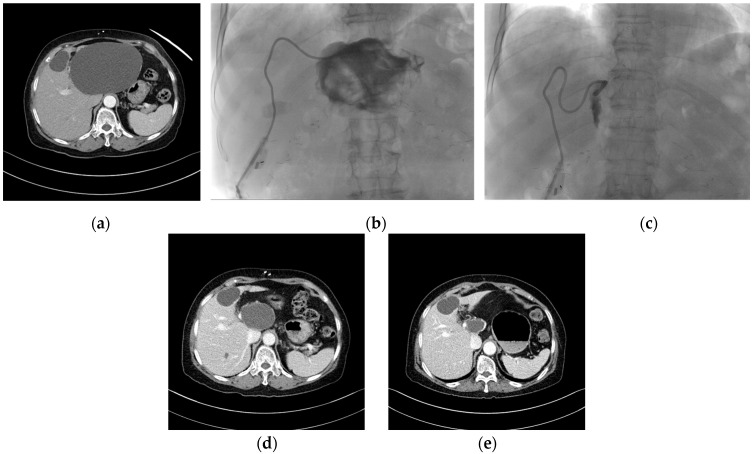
Case of incomplete alcohol sclerotherapy and natural course after treatment. (a) An enhanced CT scan performed on a 77-year-old woman presenting with early satiety revealed a large cyst measuring 14 × 10 × 13 cm maximum orthogonal cyst diameter in the left liver. The patient had a history of alcohol sensitivity. (b) After natural drainage of 1000 mL over the course of one day, tubography was performed. The cystic cavity was found to be collapsed, and contrast infusion and regurgitation proceeded smoothly. The planned injection of 100 mL of 99% alcohol was then initiated. However, at the time of injection of 40 mL, severe symptoms of alcohol intoxication manifested, which led to an immediate discontinuation of the injection and removal of the alcohol. (c) The next day, 18 mL of drainage was observed, so a second alcohol sclerotherapy was attempted, but severe pain occurred again when another 30 mL of alcohol was injected, so the alcohol was immediately removed. In the final tubography, the cystic cavity was found to be even more collapsed than before. Natural drainage was performed for 6 h, with minimal fluid drainage leading to the removal of the catheter. (d) CT scan performed after 6 months revealed the cystic cavity, measuring 6 × 5 × 6 cm in size. (e) CT scan performed after 12 months revealed that the size of the cyst was smaller (2 × 4 × 3 cm) without repeated sclerotherapy, and the cyst had shrunk to 96% of its pre-treatment size at 12 months.
